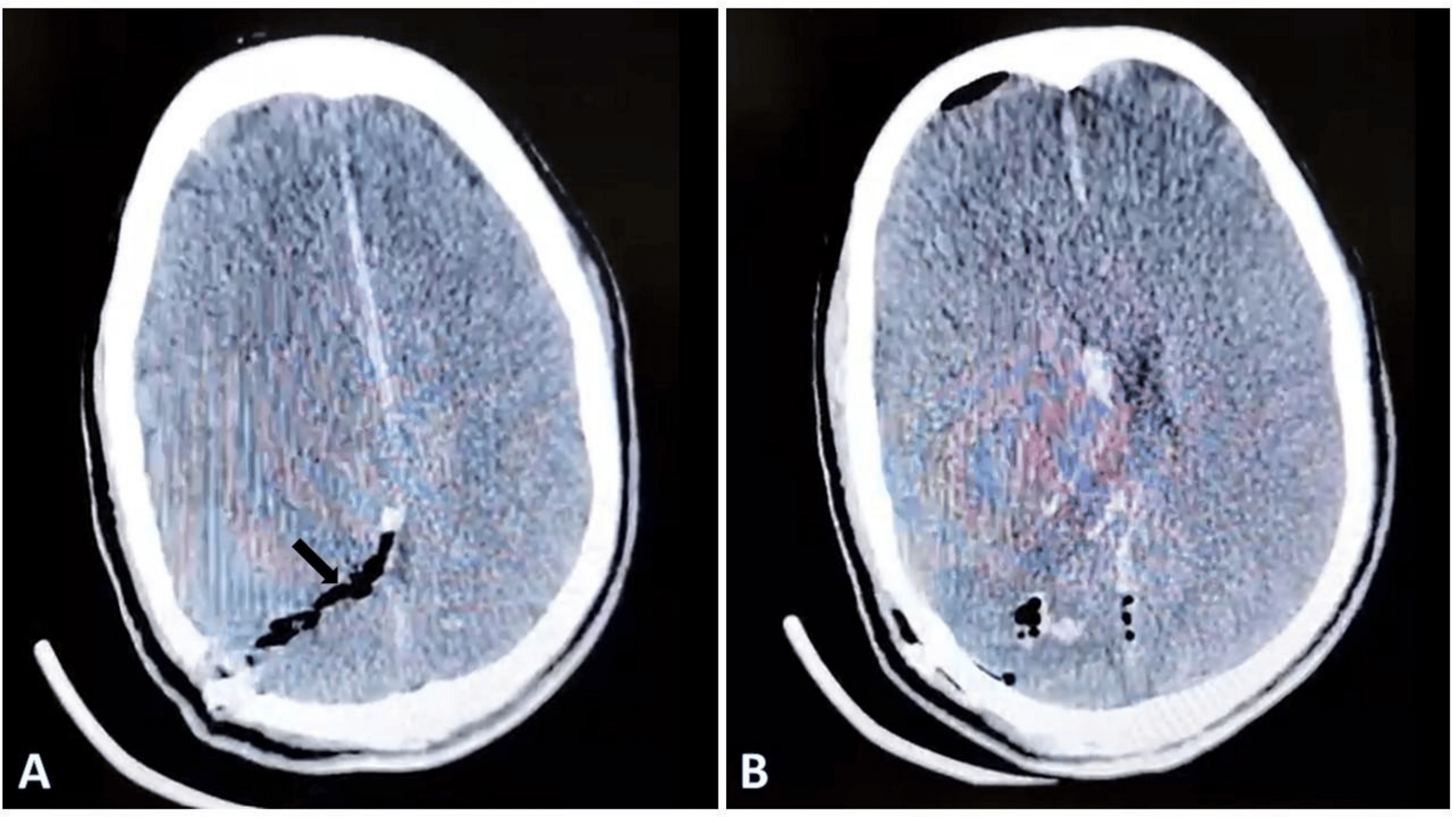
Postoperative computed tomography demonstrating intracranial changes after removal of the retained axe. (A) Axial postoperative CT scan demonstrating the postoperative tract with residual intracranial air and hemorrhagic contusion along the trajectory (arrow).
